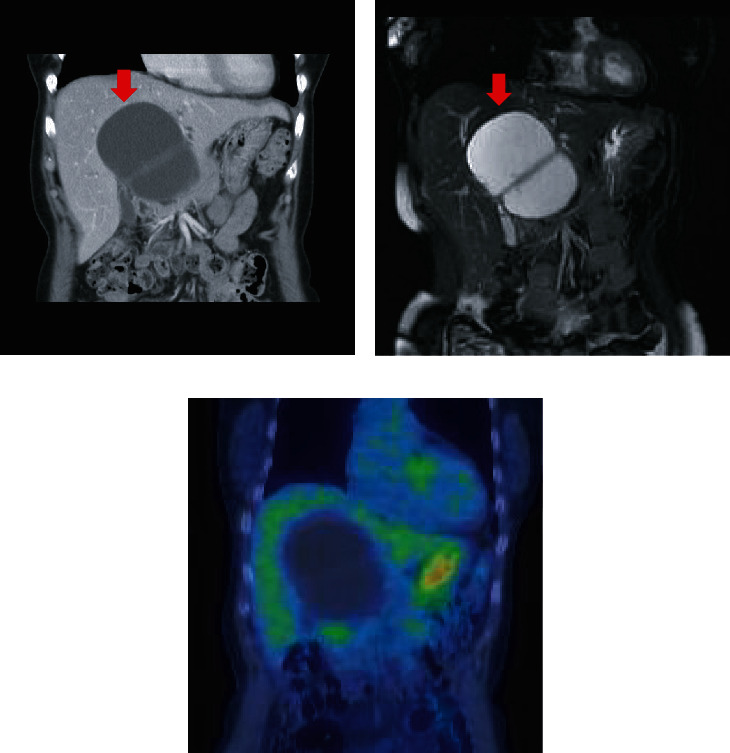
(a) Enhanced computed tomography and (b) T2-weighted magnetic resonance imaging show cystic lesions with septations arising from macrolobulations in the medial segment of the liver (red arrows). Uptake of fluorodeoxyglucose in the cystic lesions was not observed on positron emission tomography (c).
